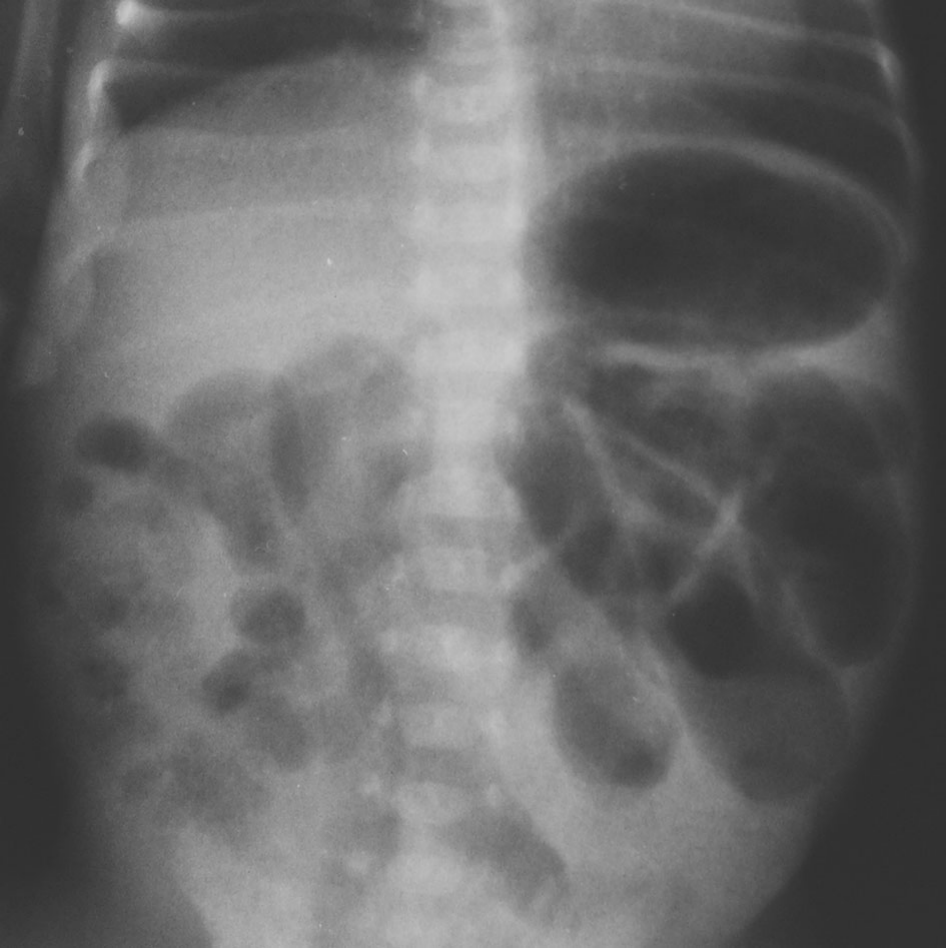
Abdominal radiograph shows diffuse gaseous intestinal distention with discrete signs suspected of pneumatosis in the lower right quadrant, six point according to DAAS scale—the example of highest variation between examiners (1, 2, 6, and 8)
